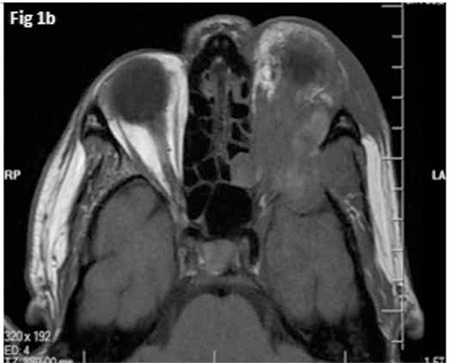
Magnetic resonance imaging image of orbital adnexal mass.
